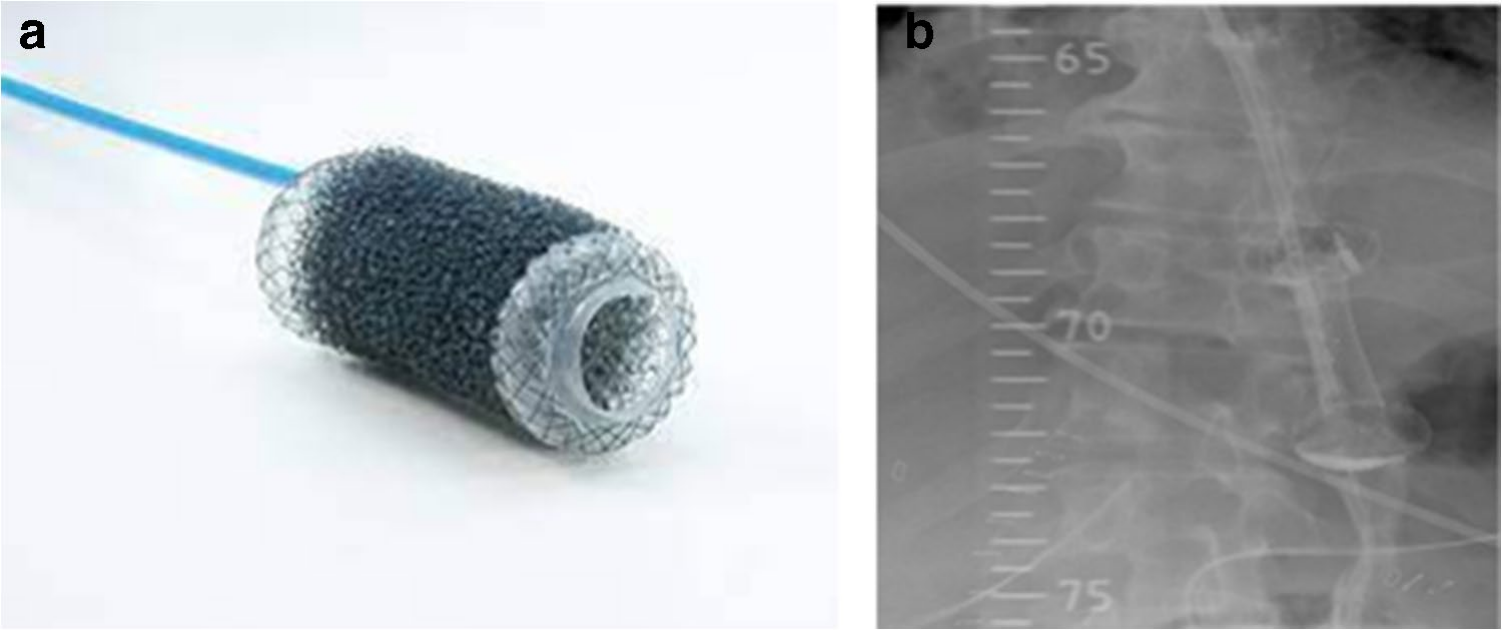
a and b The VacStent® (VAC Stent Medtec AG, Steinhausen, Switzerland) is specifically designed for EVT and combines a cylindrical PU foam with a covered SEMS. b shows a contrast radiography of patient with perforation of the esophago-gastric junction treated with a VacStent®
